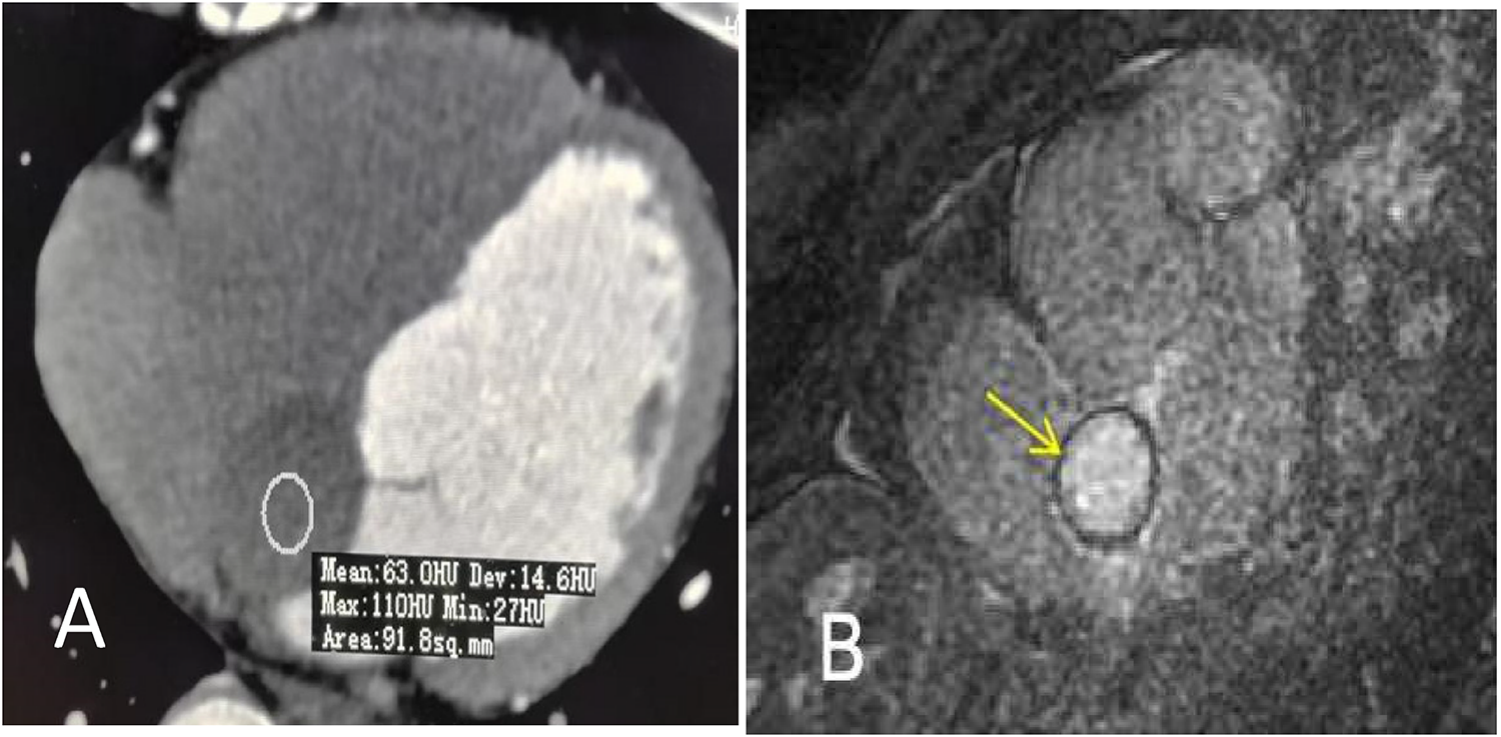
(A) Contrast-enhanced CT shows a low-intensity ovoid nodule without obvious enhancement at the interatrial septum (arrow). The mean attenuation value was approximately 63 Hu. (B) Cardiac MRI shows a round mass in the interatrial septum measuring 30 mm × 20 mm (arrow). Neither fat deposition nor late gadolinium enhancement were identified in the tumor.
